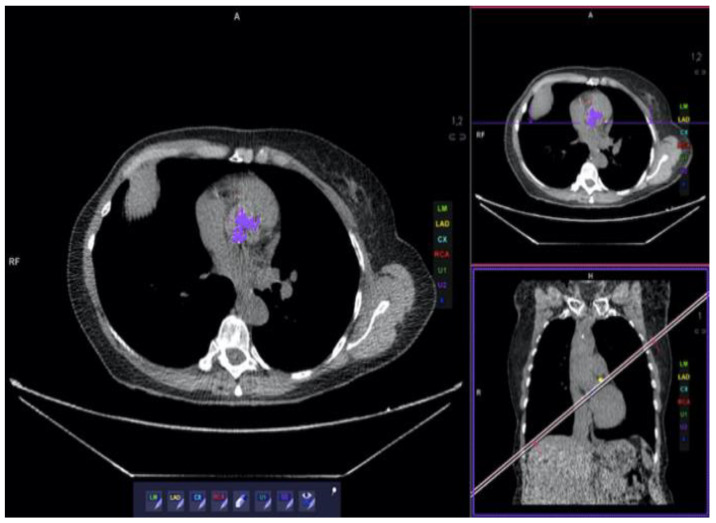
Patient with massive calcification. The calcium score was 4075. In echocardiography, severe aortic stenosis was diagnosed.
